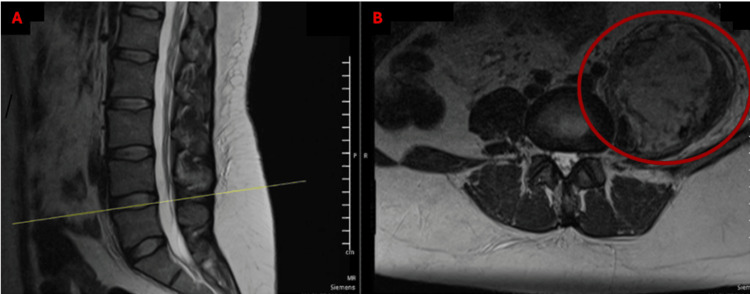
Initial iliopsoas hematoma identified on magnetic resonance imaging (MRI) Simple MRI obtained during admission in a T2 sequence, showing an intraperitoneal collection in the psoas muscle of approximately 896 cc. (A) Sagittal section; (B) Cross-section Cc: cubic centimeters
